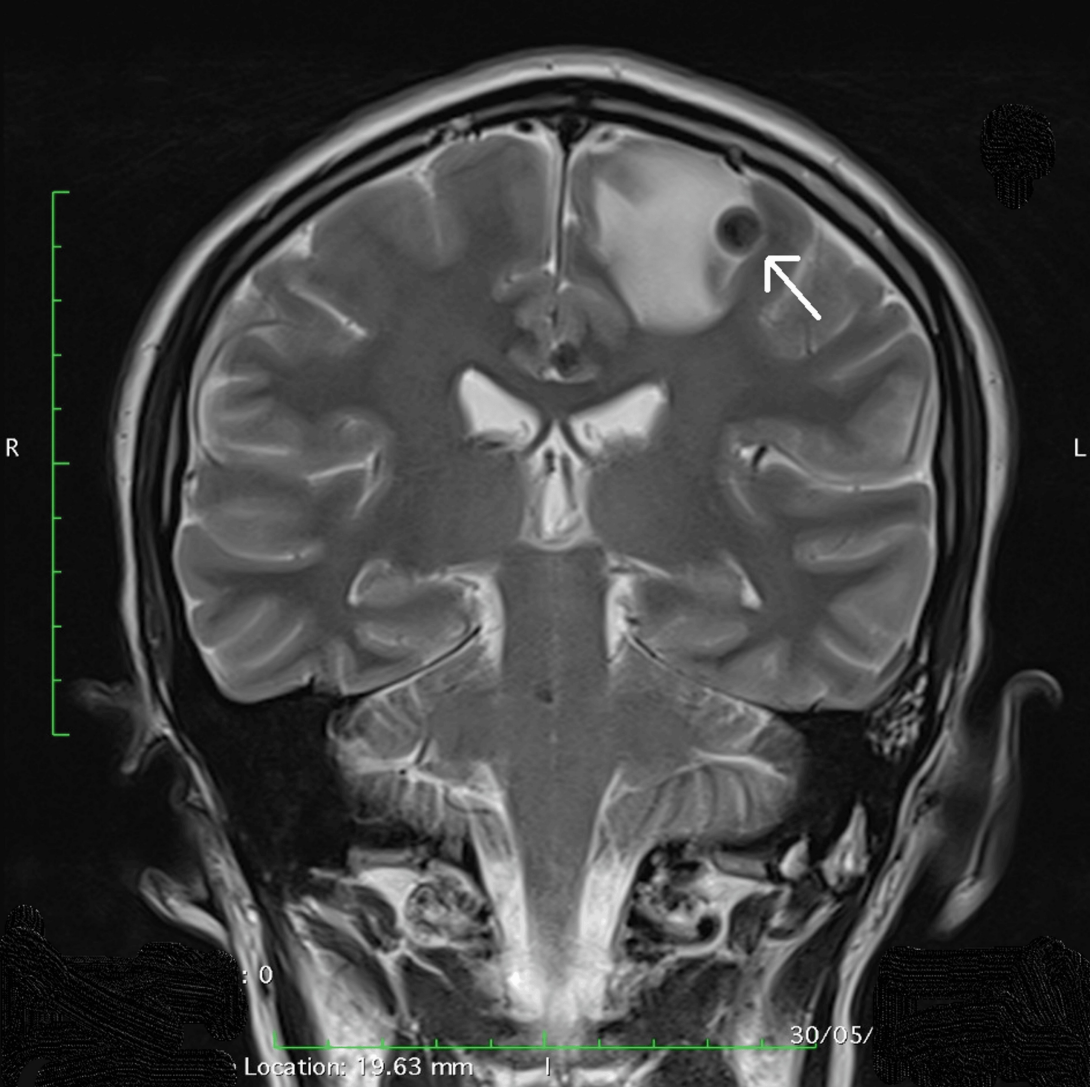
MRI of the brain, T2-weighted coronal section, showing left frontal cyst with hypointense cystic wall and significant perilesional edema (thin arrow).
